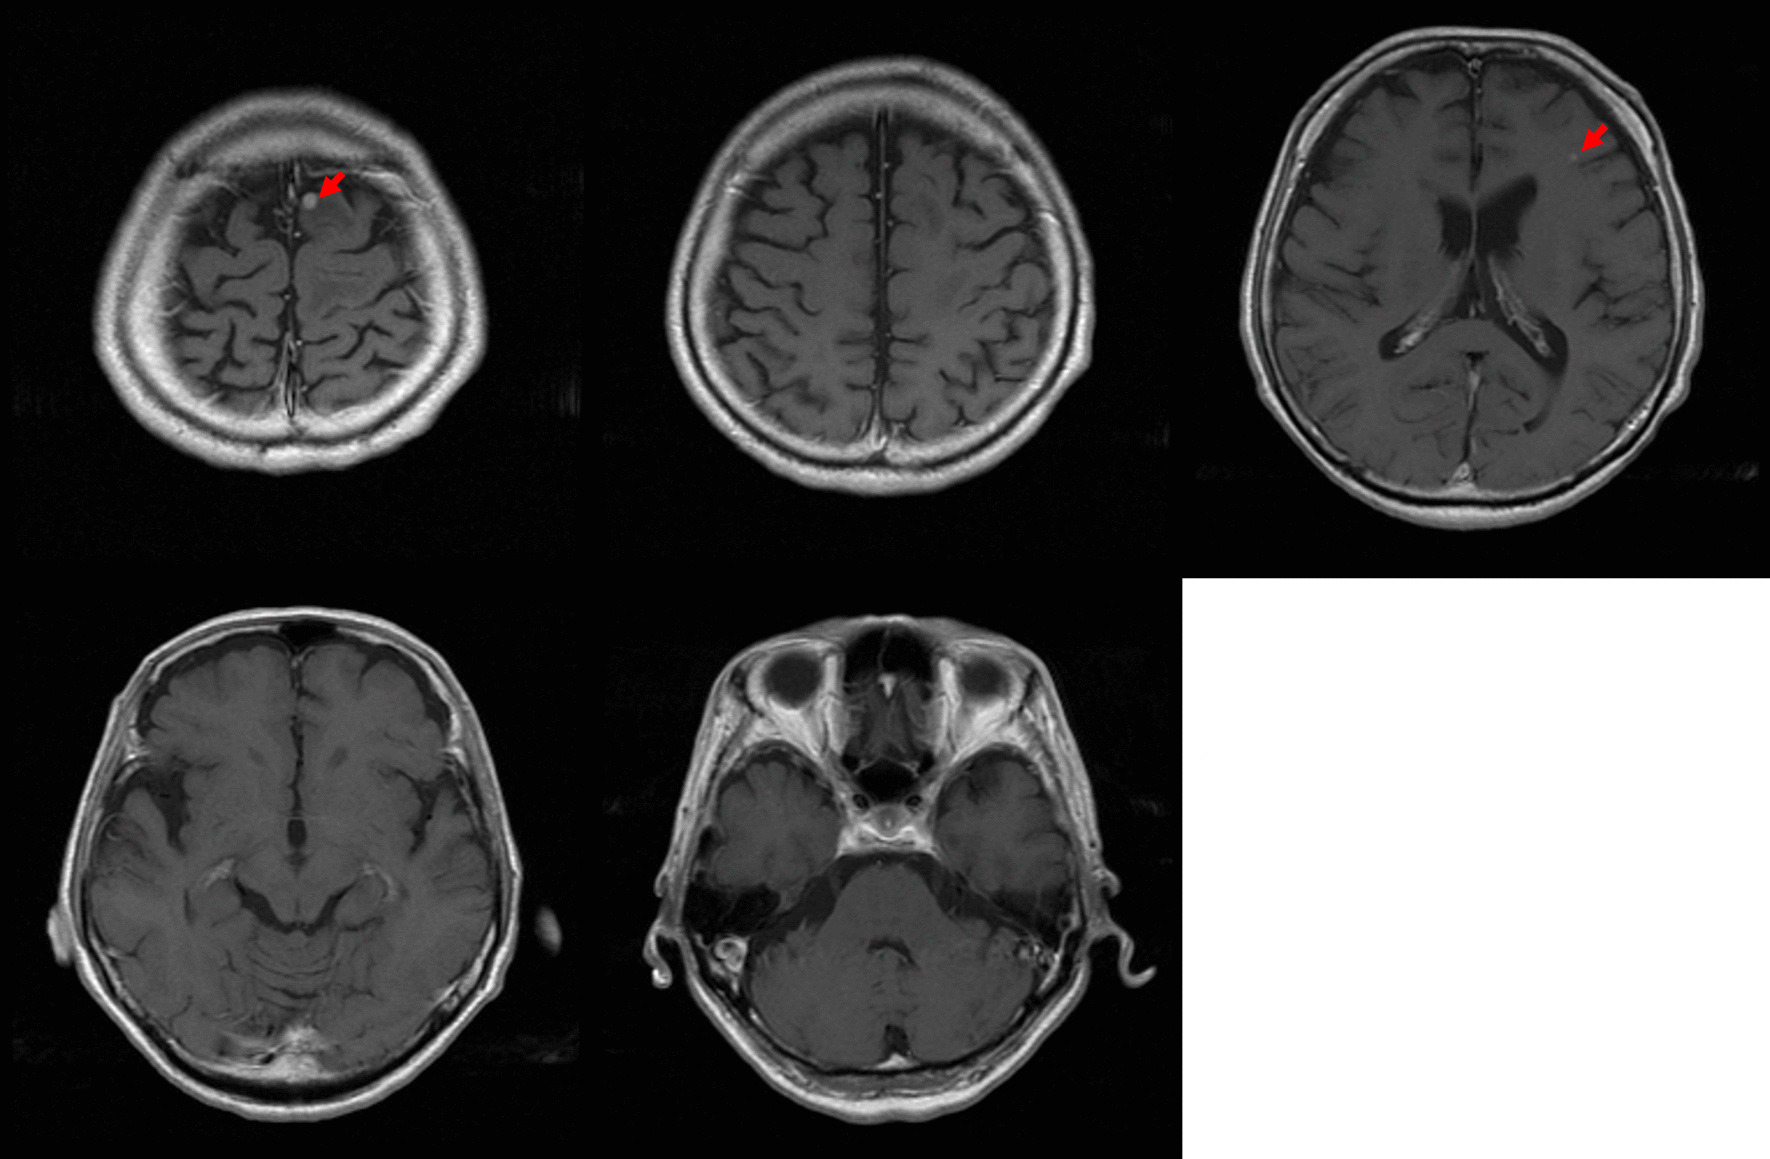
Magnetic resonance imaging for the purpose of whole-brain radiotherapy showed that almost all of the tumors had spontaneously shrunk 1 month after radiotherapy for the ninth vertebra. The two tumors in the frontal lobe had not shrunk or grown (red arrows)
